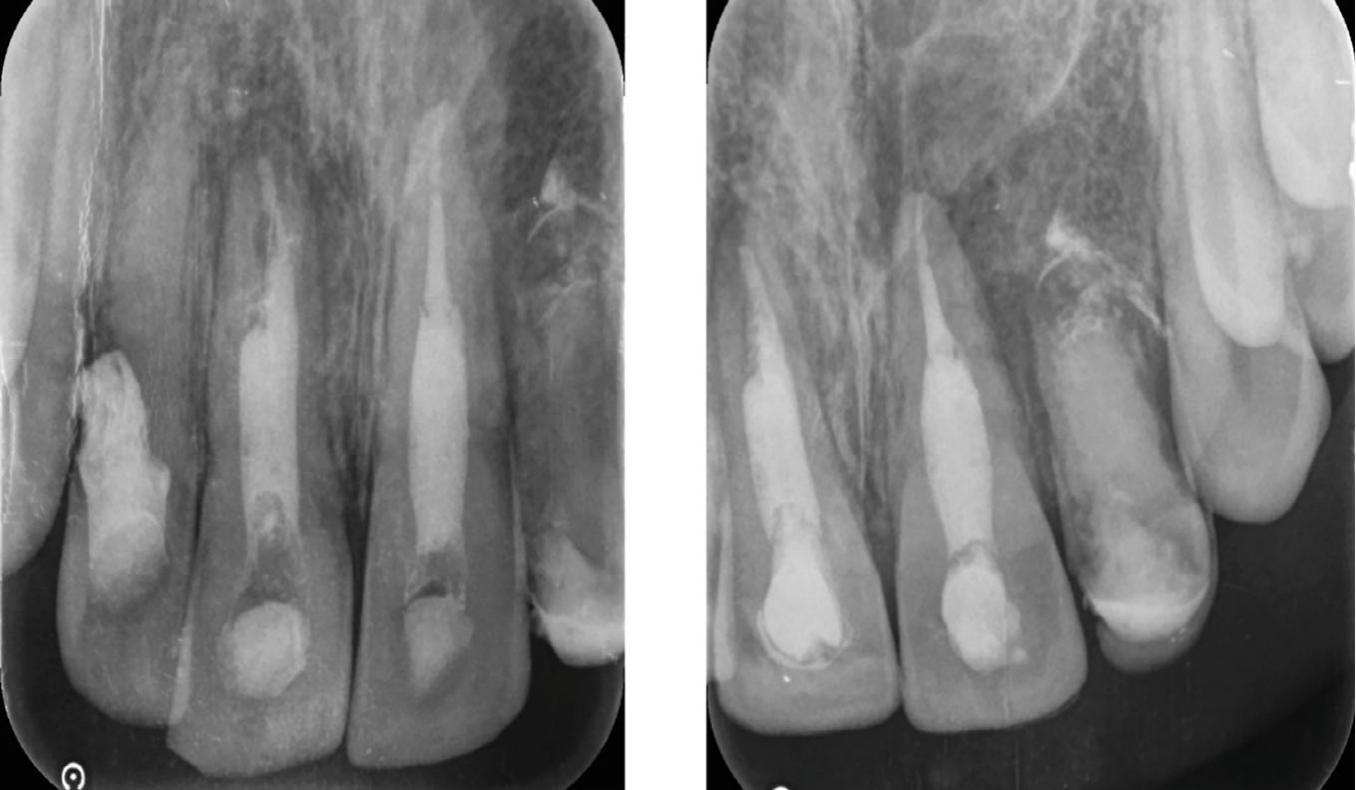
Intraoral periapical radiographs of 12 months' follow-up.
